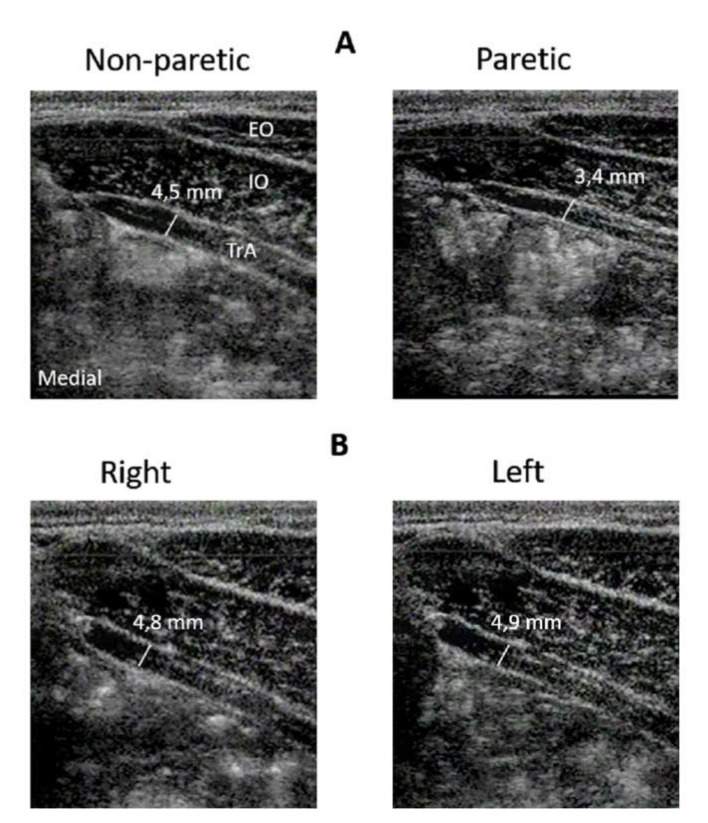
Example ultrasound images of the transversus abdominal (TrA) muscle from A. an individual with hemiparesis and B. control group from each side of the body at rest. The TrA muscle is located in this image as a layer under the external (EO) and internal (IO) oblique abdominals. Thickness measurements were made between the superficial and deep borders of the TrA muscle.
